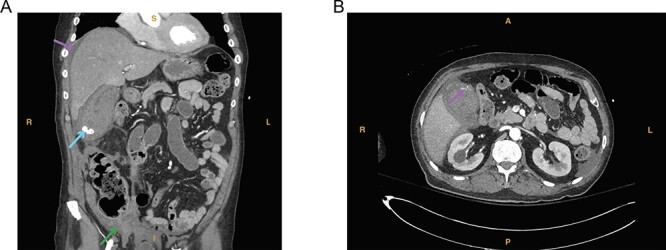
(A) Computed tomography imaging from Case 1 showing a distended gallbladder with multiple calculi (cyan arrow) as well as hyperdense intraluminal fluid (magenta arrow) with collection in the pelvis (green arrow) consistent with hemorrhage. Present in the figure, the letters S, L, I and R denote superior, left, inferior and right, respectively. (B) Computed tomography imaging from Case 1 showing the tortuous path of a cholecystic artery branch (magenta arrow) at the gallbladder fundus. Present in the figure, the letters A, L, P and R denote anterior, left, posterior and right, respectively.
